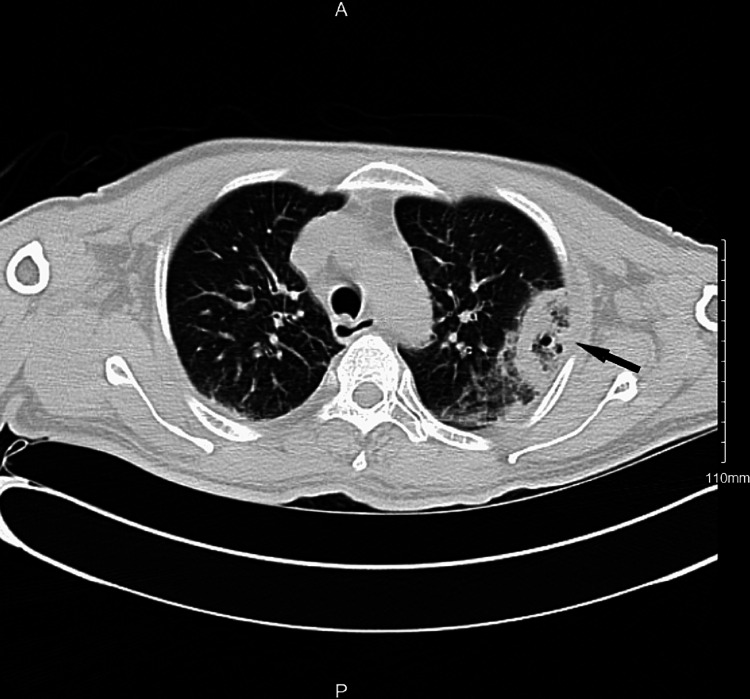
Computed tomography (CT) of chest depicting left upper lobe peripheral necrotizing lesion (arrow).
